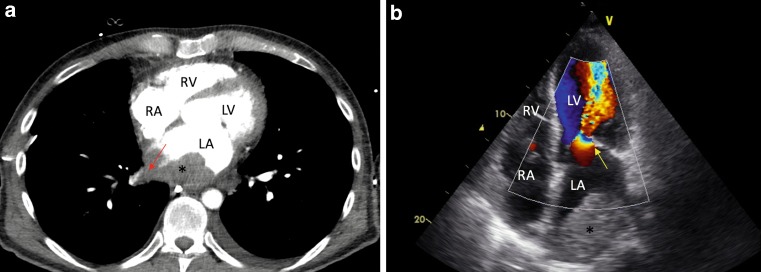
a Contrast thoracic CT scan showing a left atrial mass (*), with right inferior pulmonary vein infiltration (*red arrow*). b Apical four-chamber view from transthoracic echocardiography showing an enlarged left atrium with a giant left atrial thrombus (*). Rheumatic mitral valve with bileaflet restriction and commissural fusion resulting in severe stenosis (*yellow arrow*). *LA* left atrium, *LV* left ventricle, *RA* right atrium,* RV* right ventricle
